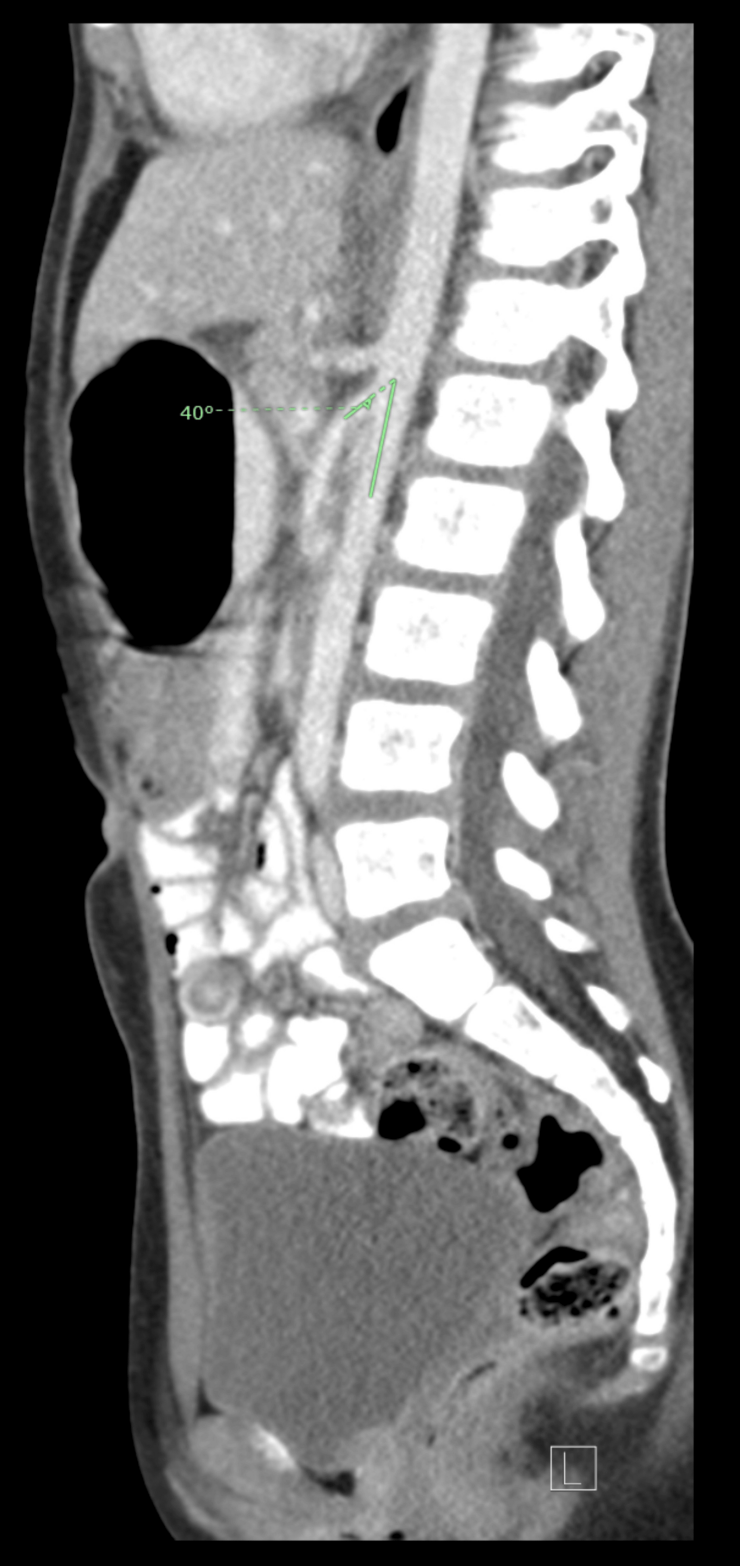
Sagittal CT showing collapsed duodenum between the aorta and SMA with narrowed angle SMA, superior mesenteric artery
